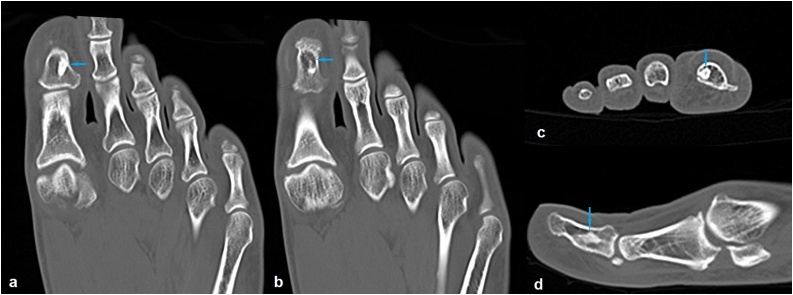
Consecutive coronal (a, b), axial (c) and sagittal (d) computed tomography images demonstrating the typical bony findings of the osteoid osteoma in the distal hallux.
